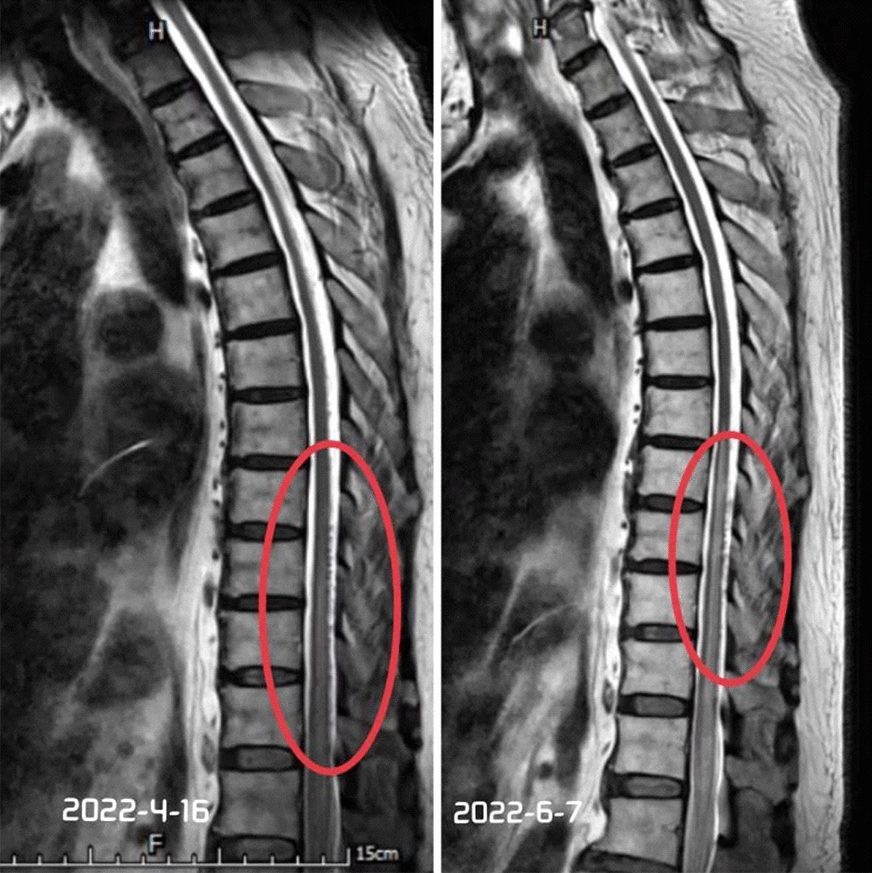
Comparison of MR images of the thoracic vertebrae before and after treatment. There were fewer vascular empty shadows on the dorsal spinal cord than before treatment
